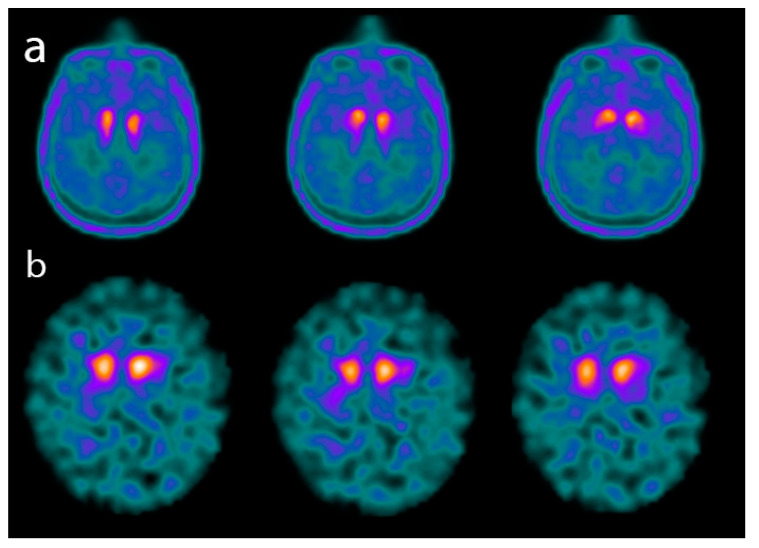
Axial images of a PET scan performed with ^18^F-Fluoro-L-dopa (FDOPA) (a) and of a single-photon emission CT (SPECT) scan performed with ^123^I-FPCIT (DATSCAN) (b) in two patients affected by advanced dementia with Lewy bodies (DLB). The images show a significant reduction in both ^18^F-FDOPA and ^123^I-FPCIT uptake in the basal ganglia.
